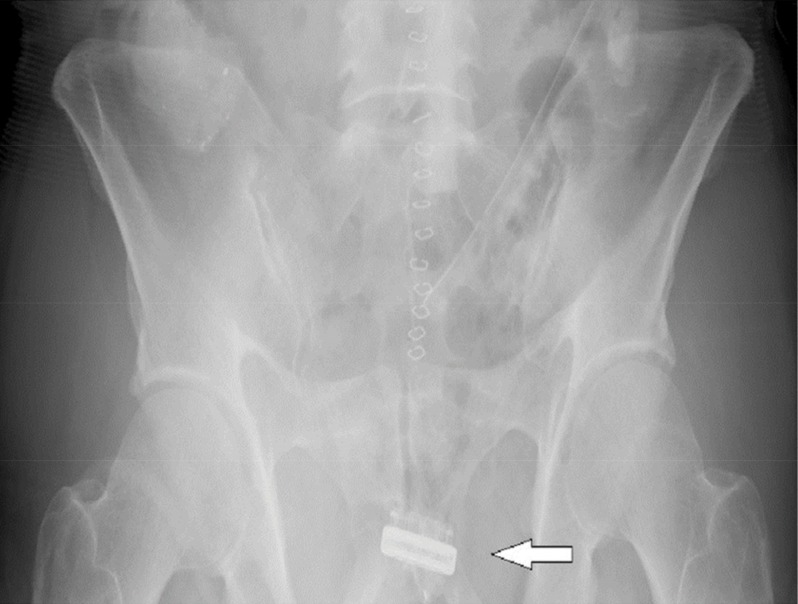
Abdominal radiographs showing the preliminary healing of anastomotic leakage after 5 days post-operation with the ring (arrow) retained in the anastomosis before the ileostomy takedown.
